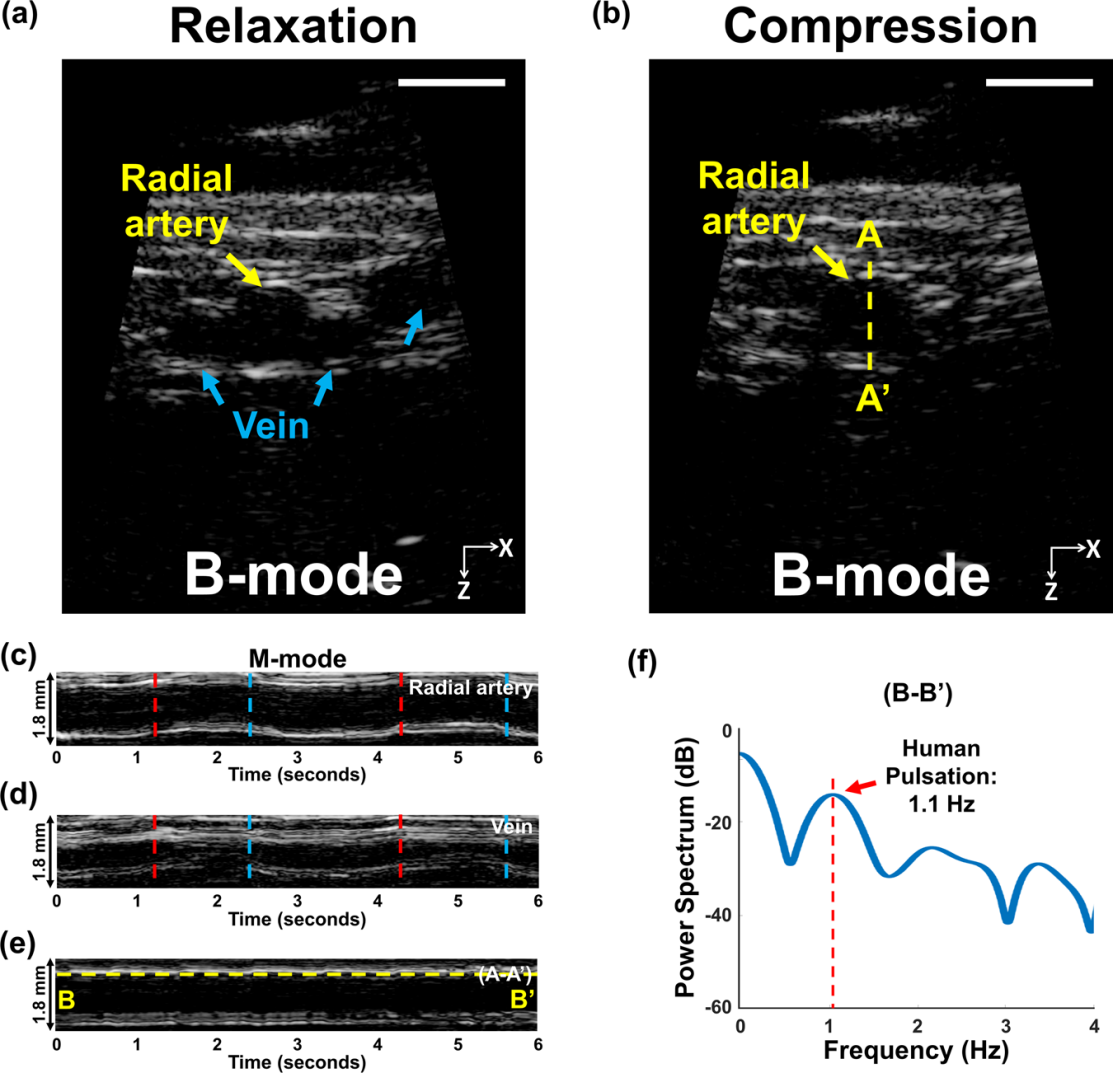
In vivo* ultrasound imaging of the wrist of a human volunteer, obtained with the MEMS-US-HH (handheld) system. Cross-sectional B-mode images of the human wrist (a) in relaxation and (b) in compression. M-mode images of (c) the radial artery, (d) the vein, and (e) the line A-A’. (f) The power spectrum of the line B-B’. All scale bars are 3 mm. Red and blue lines in (c,d) indicate compression and relaxation, respectively.
